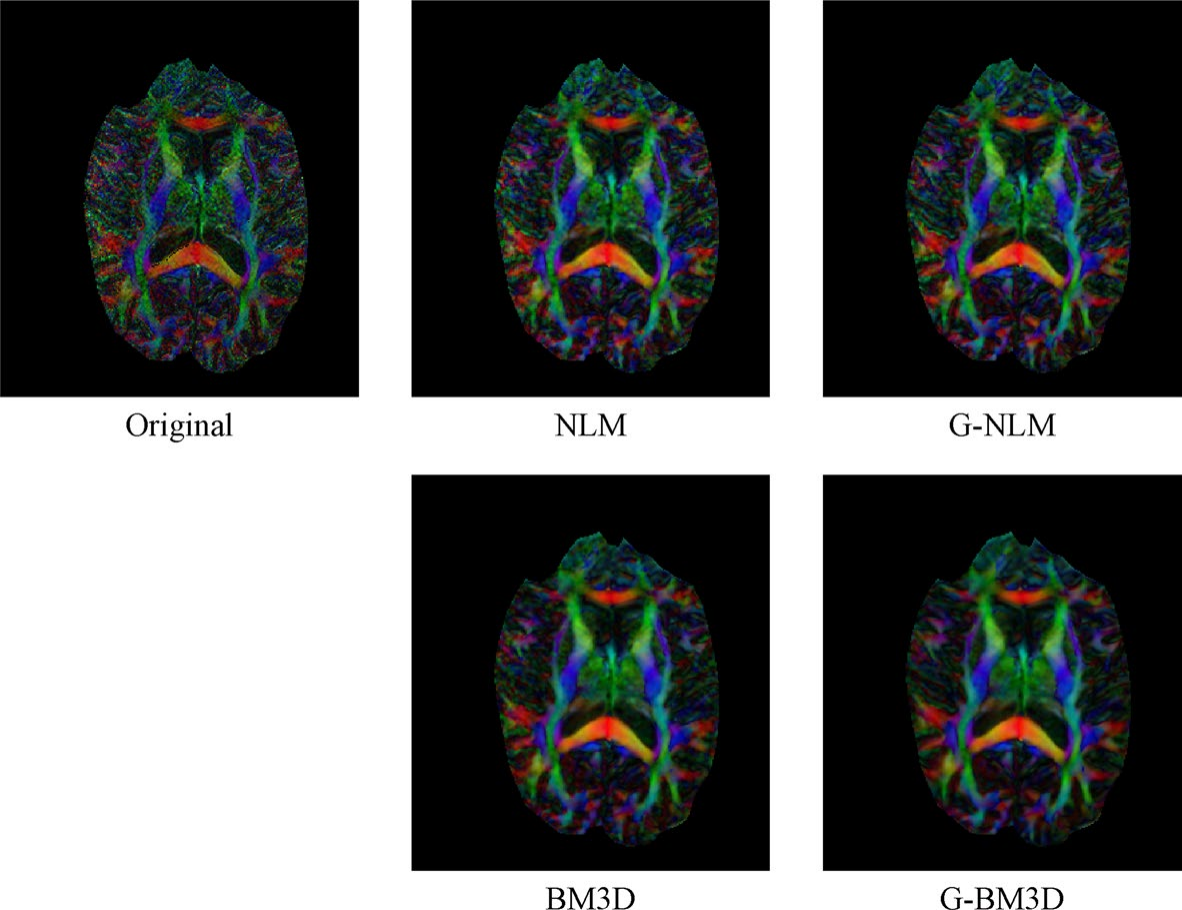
Denoising results for the principle direction weighted with FA of the DWI dataset
